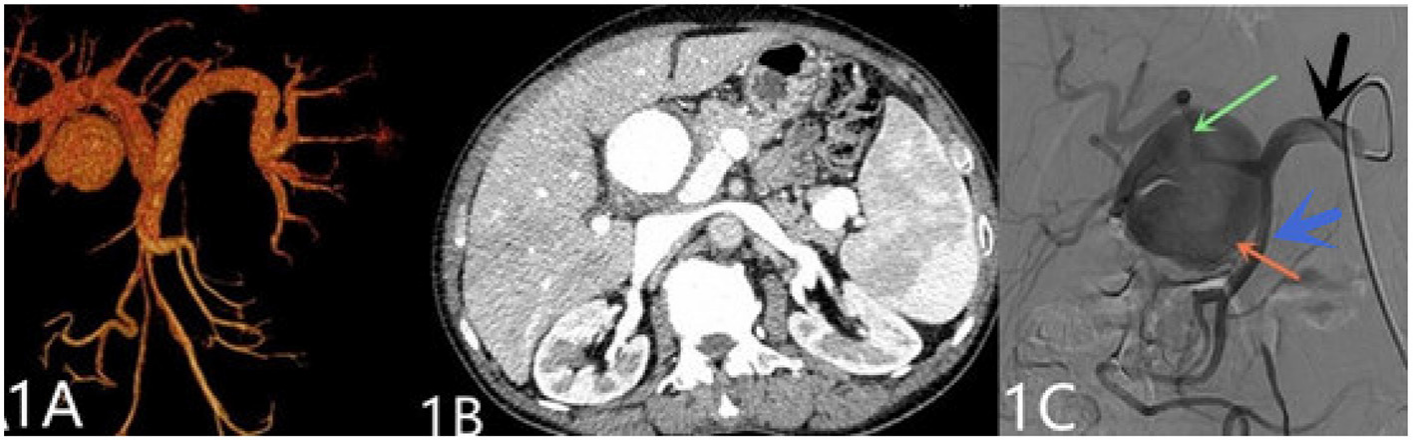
(A) Shows the pre-operative three-dimensional CT imaging of the hepatic pseudoaneurysm. (B) Displays the hepatic pseudoaneurysm shown by CT in the pre-operative patient, with a maximum cross-section of about 55 mm × 46 mm. (C) Shows the hepatic pseudoaneurysm shown by hepatic arteriography; the red arrow indicates the hepatic artery pseudoaneurysm; the green arrow indicates the proper hepatic artery; the black arrow points to the common hepatic artery; and the blue arrow points to the gastroduodenal artery.
